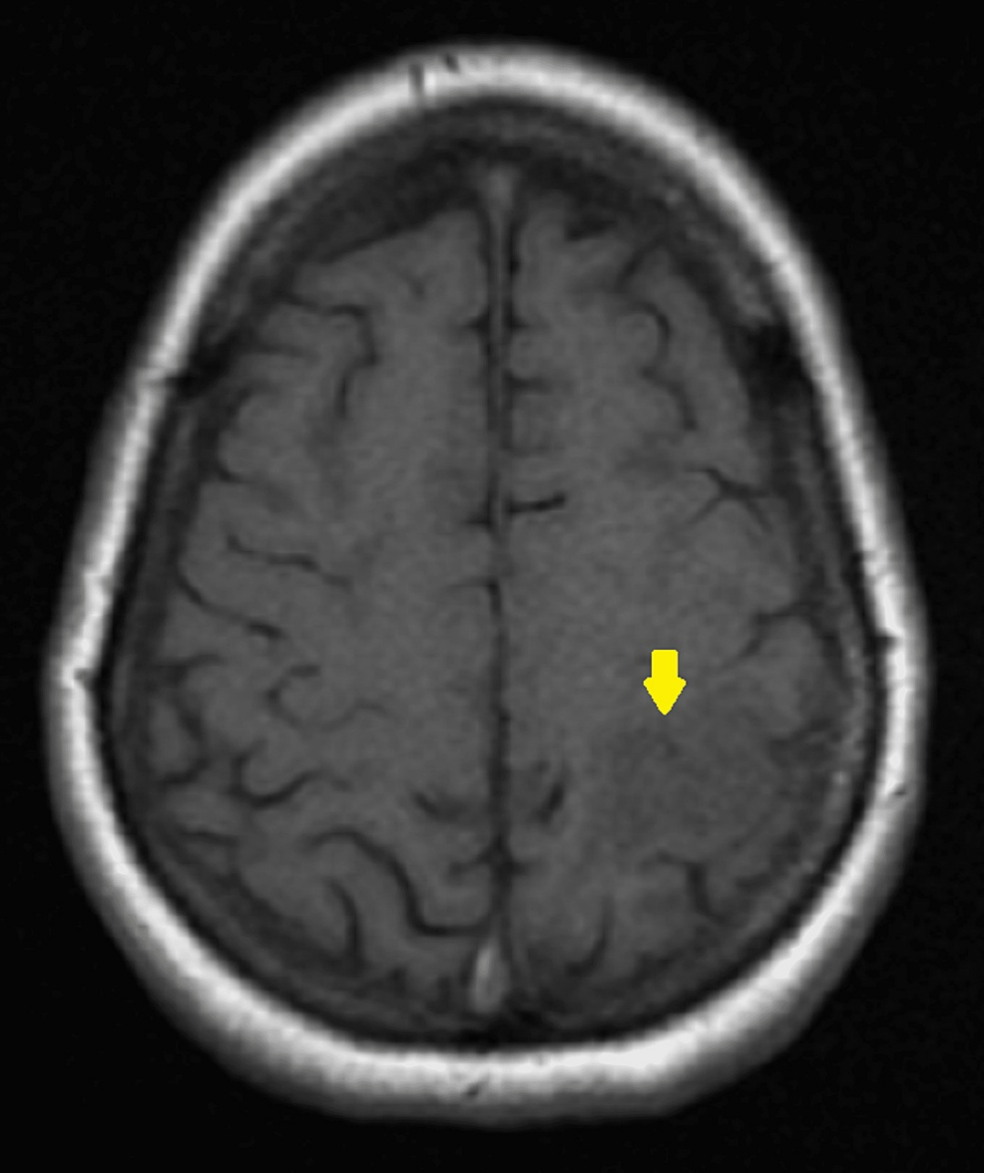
Axial view of T1 weighted MRI depicting hypointensity in left parietal lobe MRI, magnetic resonance imaging
